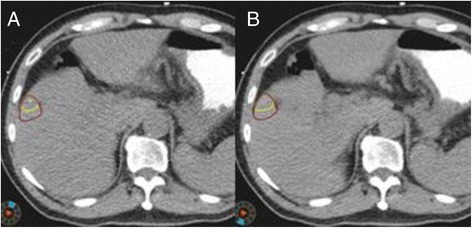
DR improves the determination of GTV contours obtained from 4D CT images (on axial view). A. CT_00_ respiratory phase of 4D CT image. B. CT_50_ respiratory phase of 4D CT images. Yellow contours: GTVs before DR; red contours: GTVs after DR.
